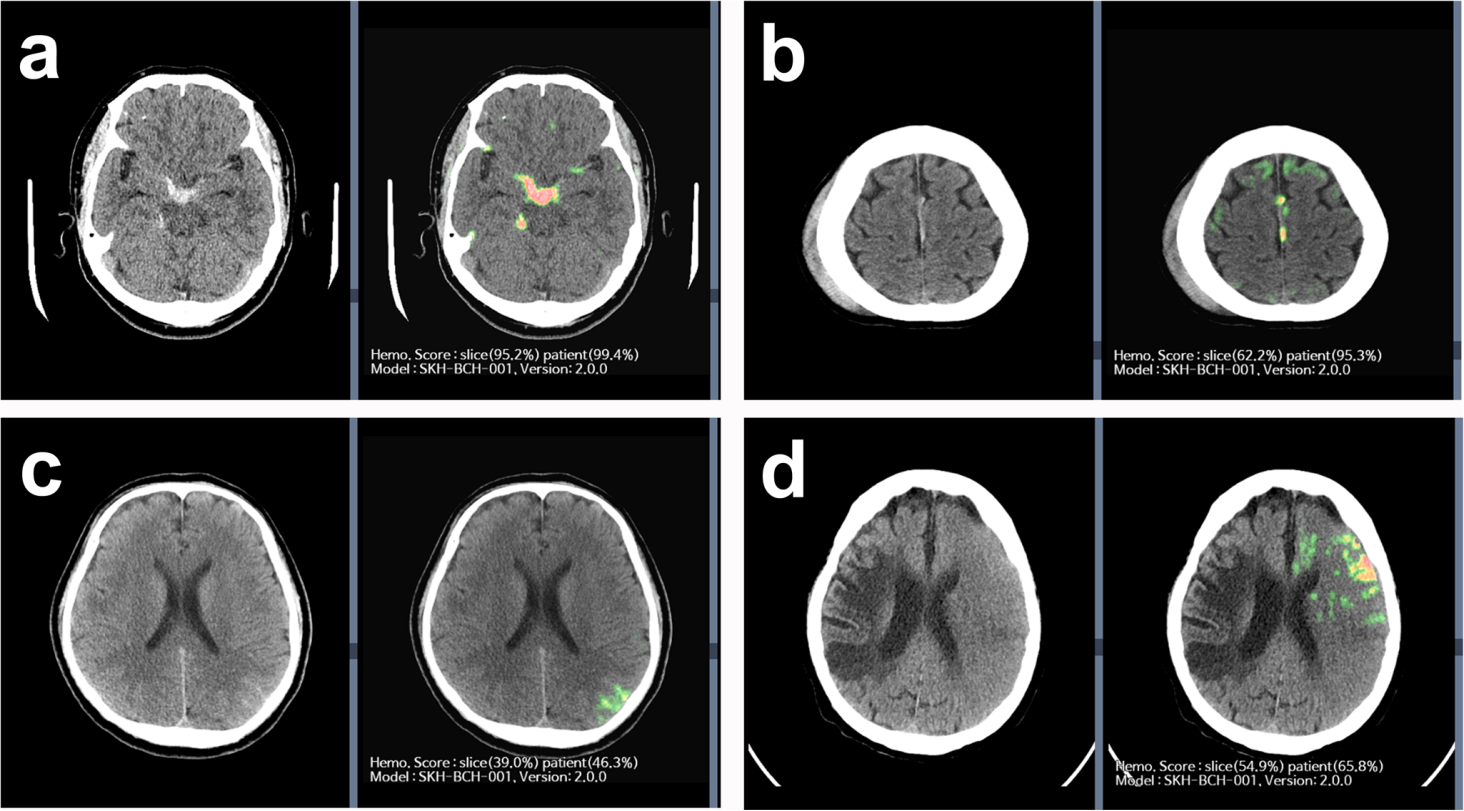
Representative images of AIH detection. a AI-assisted brain CT revealed probable AIH location as the basal cistern and right ambient cistern. AI-assisted brain CT provided AIH probability scores in a slice-wise (95.8%) and patient-wise (99.4%) manner. All nine reviewers agreed with the AIH diagnosis for both AI-unassisted and AI-assisted interpretations. b AI-assisted brain CT revealed the probable AIH location as the left side of the falx. AI-assisted brain CT provided the AIH probability scores in a slice-wise (62.2%) and patient-wise (95.3%) manner. For interpretation without AI assistance, one reviewer (non-radiologist physician) missed this case of AIH on the left side of the falx. All nine reviewers agreed with the AIH diagnosis for both AI-unassisted and AI-assisted interpretations. c AI-assisted brain CT revealed probable AIH location as the left parietal sulci. AI-assisted brain CT provided AIH probability scores in a slice-wise (39.0%) and patient-wise (46.3%) manner. For interpretation without AI assistance, two-thirds of the reviewers (three non-radiologist physicians, two board-certified radiologists, and one neuroradiologist) missed this case of AIH in the left parietal sulci. With the use of AI assistance, these six reviewers were able to correctly revise their decisions. d AI-assisted brain CT revealed the probable AIH location as the left frontal area. AI-assisted brain CT provided the AIH probability scores in a slice-wise (54.9%) and patient-wise (65.8%) manner. For the interpretation without AI assistance, one-third of the reviewers (one non-radiologist physician and two board-certified radiologists) reported it as an AIH. With the use of AI assistance, an additional one-third of the reviewers (one non-radiologist physicians, one board-certified radiologist, and one neuroradiologist) reported this as an AIH. However, the subtle hyperattentuating lesion in the left frontal area was due to the beam-hardening artefact of the skull.
